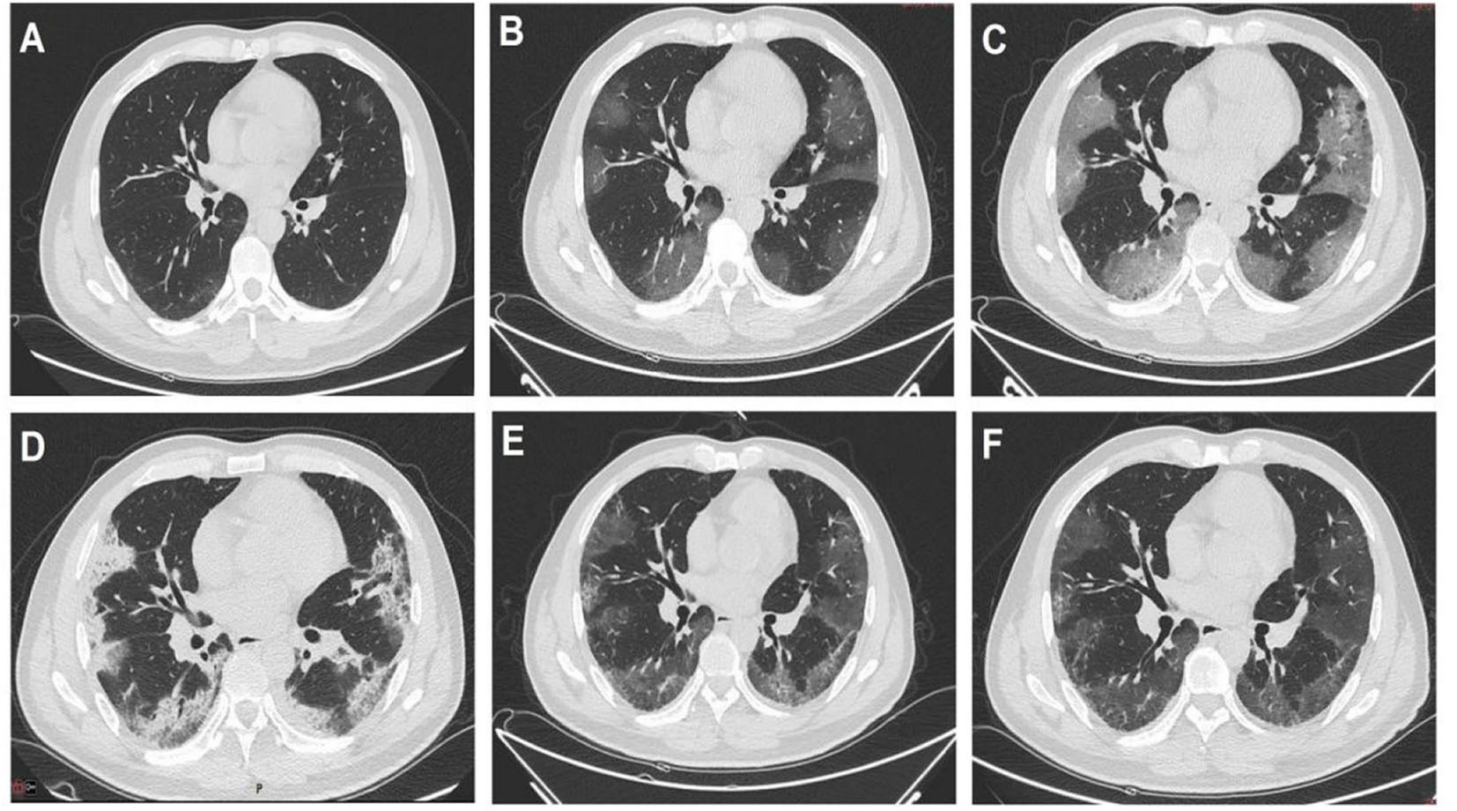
Dynamic change shown on the chest computed tomography manifestations of the patient. (A) On January 30, only small ground-glass opacity (GGO) was seen. (B) On February 4, the GGO had enlarged in both lungs and was peripherally distributed. (C) On February 9, the infiltration had increased further. (D) On February 17, the lesion was consolidated and fibrosis occurred. (E) On March 1, the lesion absorbed significantly. (F) On March 10, only a small area of GGO and fibrosis remained.
